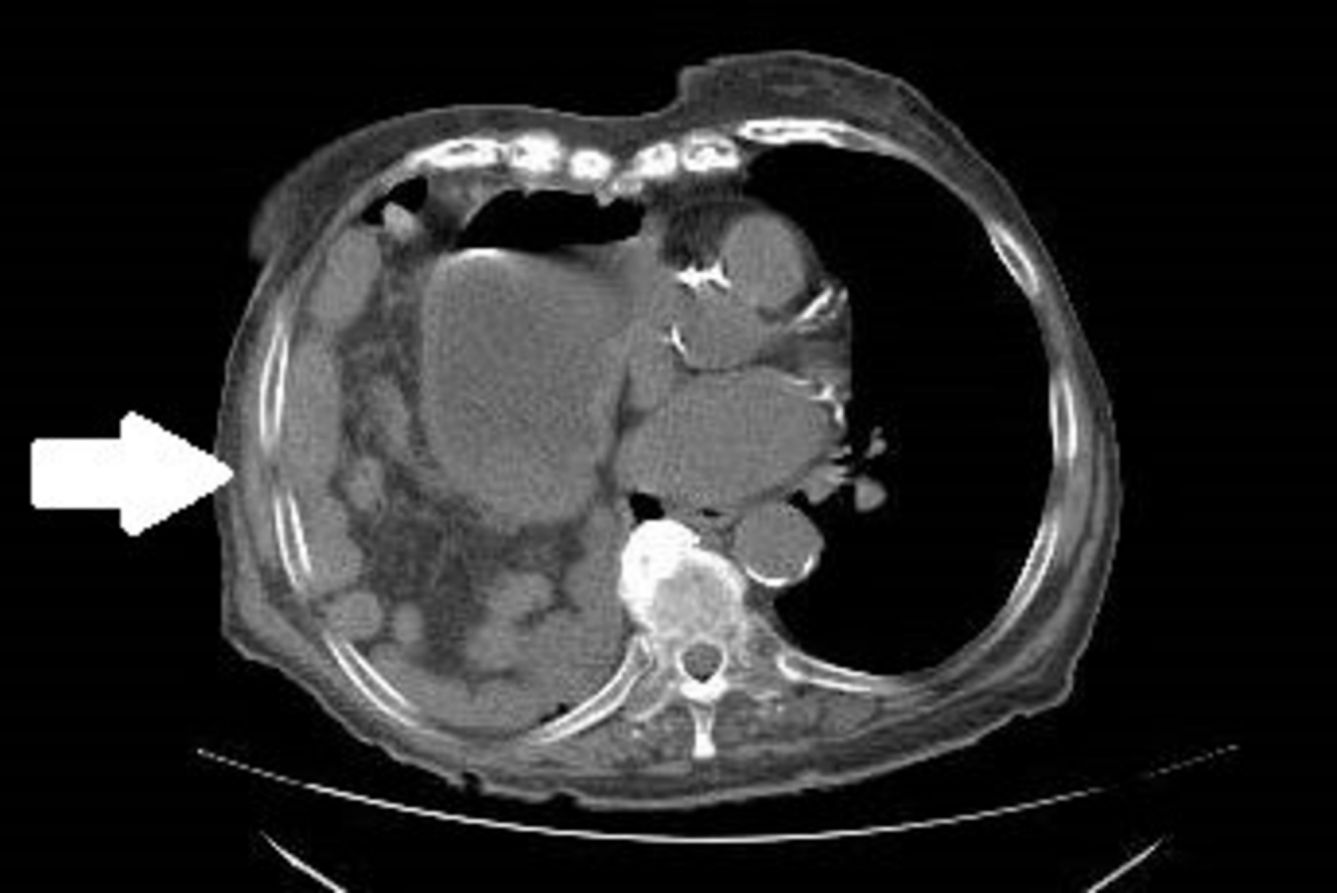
Axial view computed tomography on admission; arrow indicating stomach and intestinal contents in the thoracic cavity
